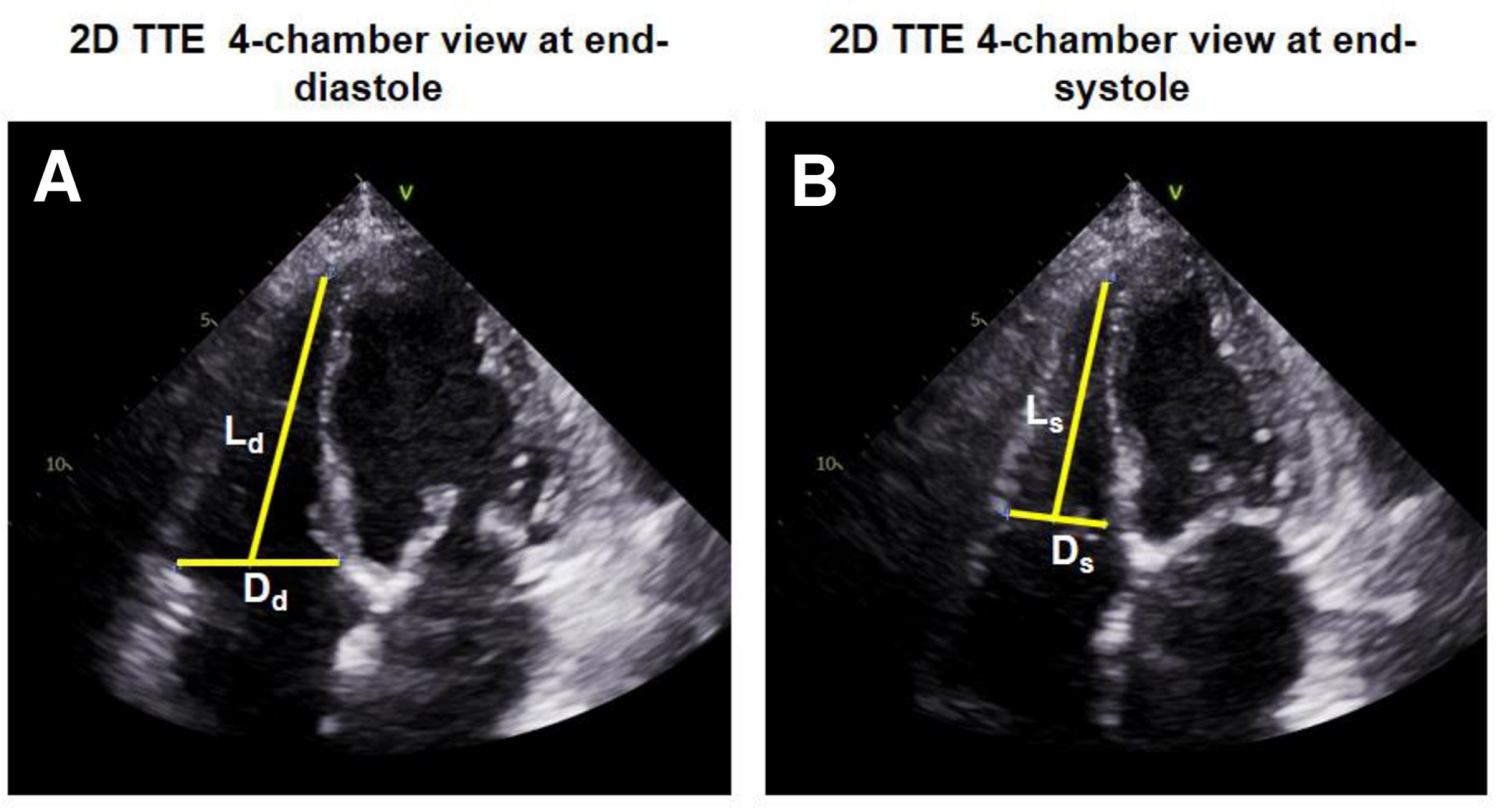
Linear dimensions measured in 2D TTE apical 4-chamber views for calculation of RV volumes and function by the proposed cone-pyramid formula (CPF).
